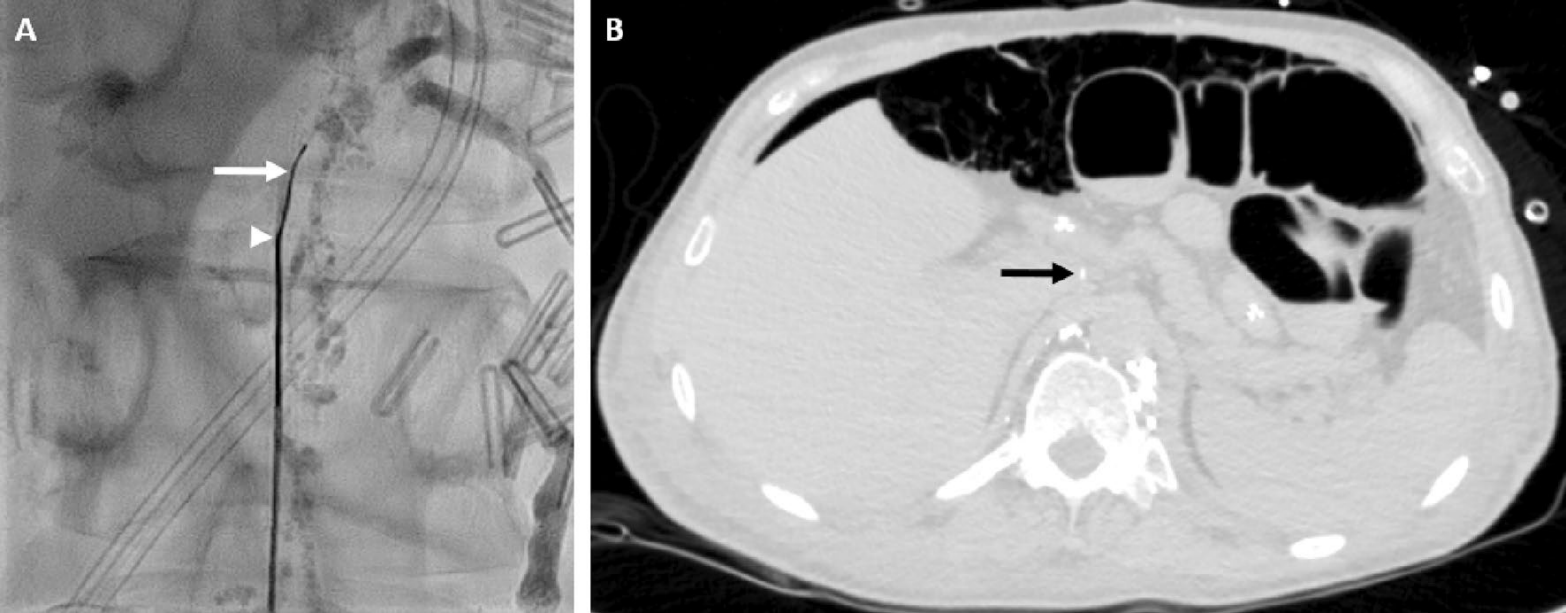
55-year-old man with intractable chylothorax after oesophagectomy. The intra-interventional fluoroscopic image (A) shows distension of the colon in the upper abdomen so that transcolonic puncture was necessary to access the target retroperitoneal lymphatics (tip of fine needle: white arrow head; micro-wire introduced into lymph vessel: white arrow). Post-interventional CT (B) showed free air in the abdomen after transcolonic puncture. The puncture tract can faintly be identified (black arrow). Antibiotics were started immediately after the intervention. The post-interventional course was uneventful without complications from transcolonic puncture. Chylous drainage ceased immediately after the intervention.
